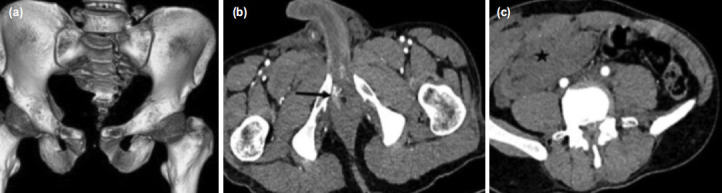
a) CT abdomen with pelvis, volume rendered image reveal fracture pattern identical to that seen on radiographs. (b) CT angiography reveal a small blob of contrast extravasation (black arrow) seen in right side of corpora cavernosa close to cavernosal branch of internal pudendal artery, (c) and hematoma (asterix) in right anterior abdominal wall and underlying part of peritoneal cavity.
